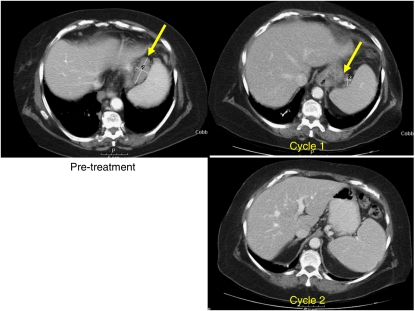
Resolution of a gastrohepatic mass in a patient with transformed DLBCL following oral vorinostat therapy. CT scans were taken before treatment and after one and two cycles of oral vorinostat.
